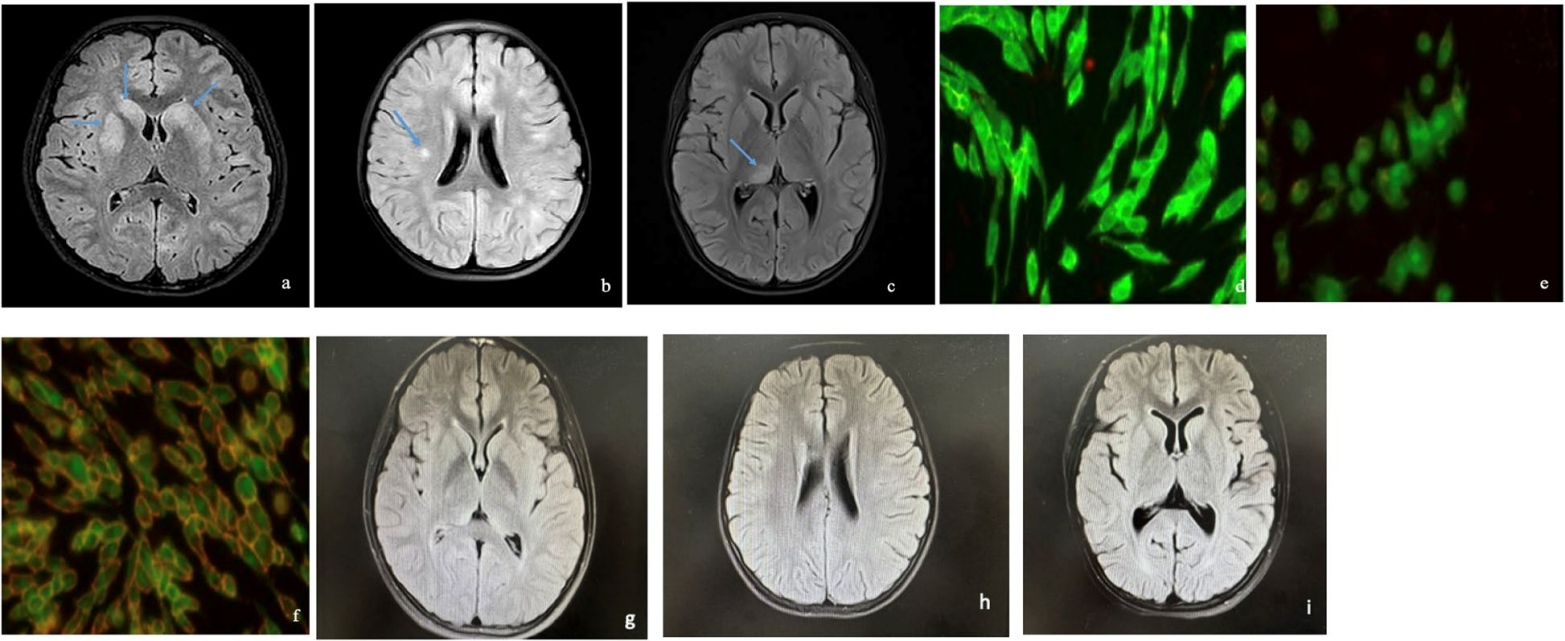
a patient 1. Axial FLAIR demonstrating hyperintensities on the bilateral caudate nucleus and lenticular nucleus; b patient 2. Axial FLAIR demonstrating hyperintensity on right centrum semiovale; c patient 3. Axial FLAIR demonstrating hyperintensity on right dorsal thalamus. d patient 1. MOG-IgG (green) in serum was detected by cell transfection assay; e patient 2. MOG-IgG (green) in serum was detected by cell transfection assay; f patient 3. MOG-IgG (green) in serum was detected by cell transfection assay; g patient 1. cranial MRI scan was normal 8 months after discharge; h patient 2. cranial MRI scan was normal 6 months after discharge; i patient 3. cranial MRI scan was normal 7 months after discharge. Ig: Immunoglobulin; MOG: Myelin oligodendrocyte glycoprotein
